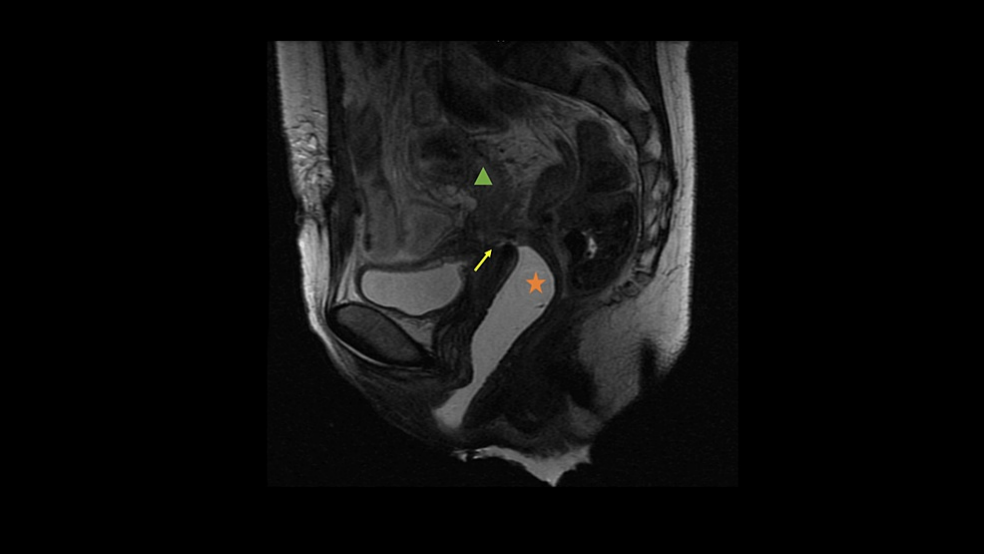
MRI showing a fistula between the sigmoid colon and vagina Green triangle: Sigmoid colon, Orange star: Vagina, Yellow arrow: Fistula
